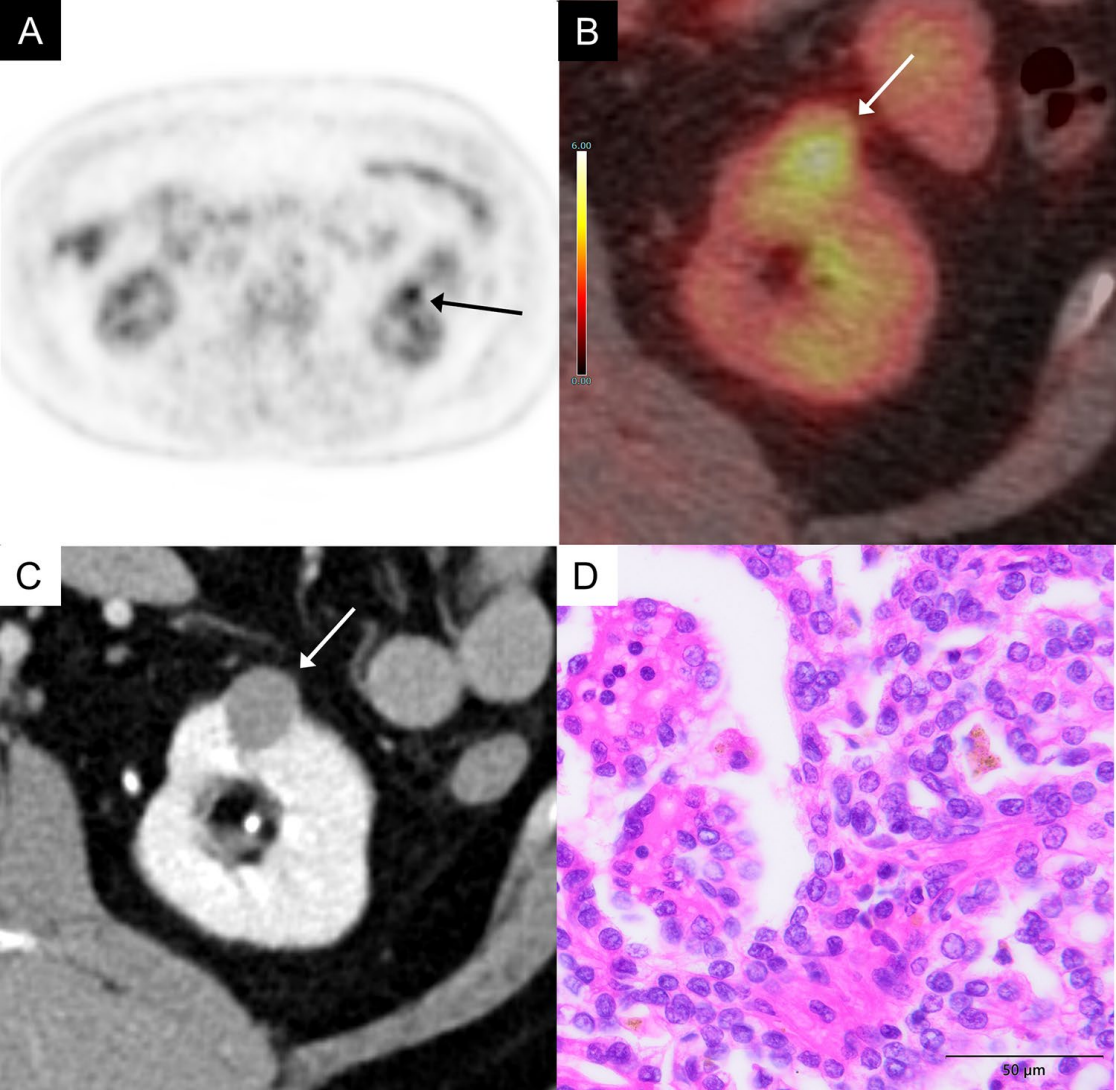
A 45-year-old man with a papillary renal cell carcinoma (WHO/ISUP grade 2) of the left kidney. A Axial FDG-PET image (gray scale) shows moderate FDG uptake in a mass of the left kidney (arrow). B Axial FDG-PET/CT image shows moderate FDG uptake in a mass of the left kidney (arrow), showing an SUVmax 5.4, while normal renal cortex shows an SUVmax 2.8. C Axial contrast-enhanced CT in the nephrographic phase shows a poor enhancement in the mass (arrow). D The tumor exhibits predominantly tubular and cribriform structures, with a papillary pattern in several regions. Conspicuous and eosinophilic nucleoli are visible only at a high-power view (× 400)
